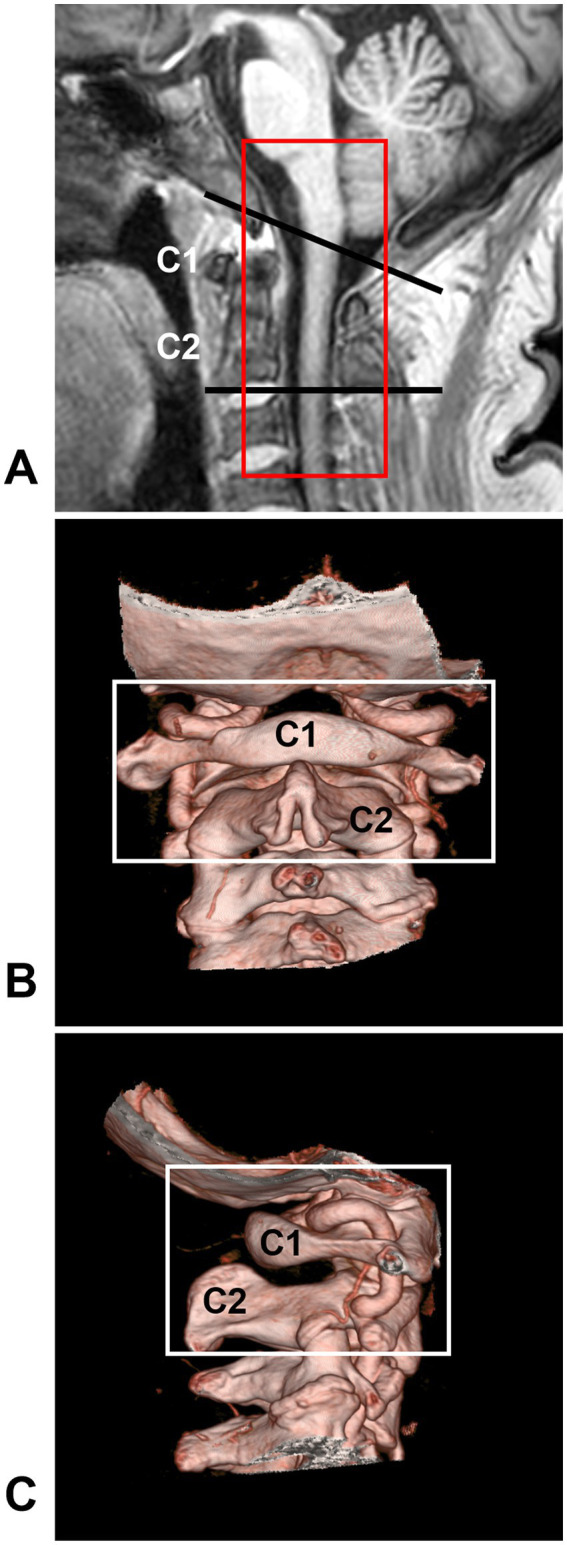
CCJ region definition. (A) MRI showing that the CCJ region was located between two black lines and formed by the occipital bone and C1-2 vertebrae. The red frame indicates the area often involved in CCJ AVF. (B,C) CTA showing the bony CCJ region (frames). AVF, arteriovenous fistula; C1-2, first and second cervical vertebrae; CCJ, craniocervical junction; CTA, computed tomography angiography; MRI, magnetic resonance imaging.
